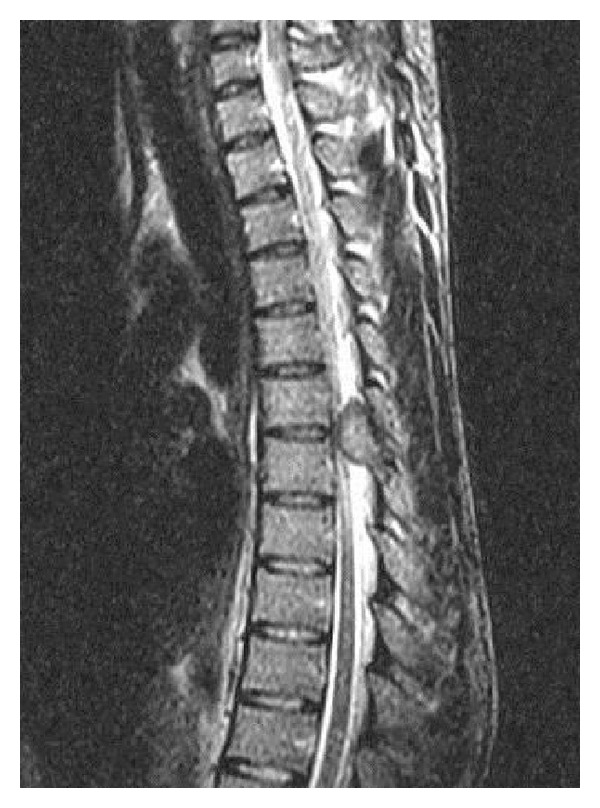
Sagittal T2-weighted images show expansive mass lesion involving the posterior elements of T6 and T7 vertebrae.
